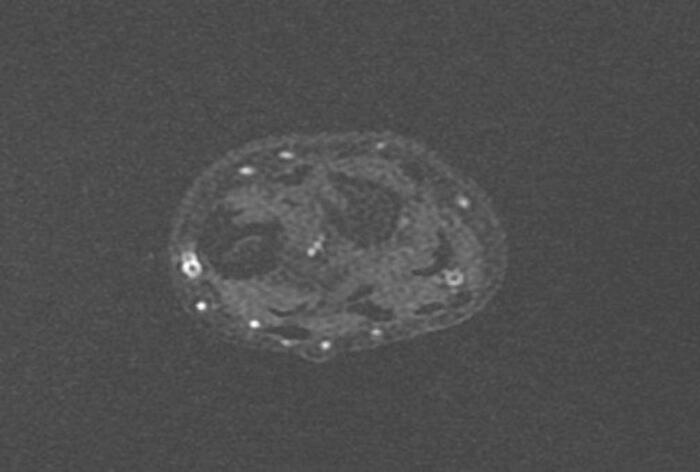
Postoperative MRI indicates the total removal of the FCD (axial view).
